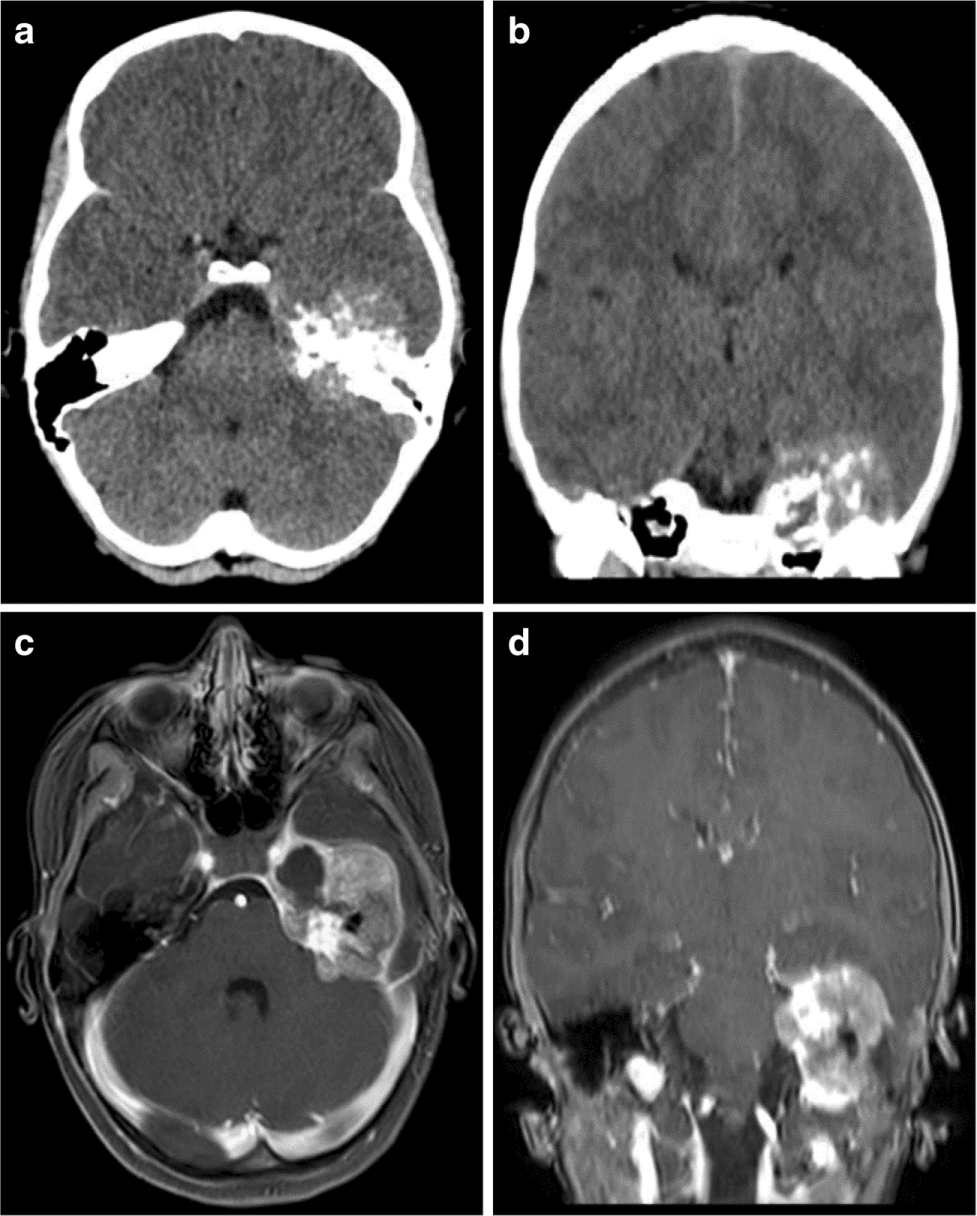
a Images from Case 4 of a 9-year-old female presenting with a left petrous apex tumor. Axial non-contrast CT. b Coronal non-contrast CT. c Axial T1-weighted post-contrast MRI. d Coronal T1-weighted post-contrast MRI
